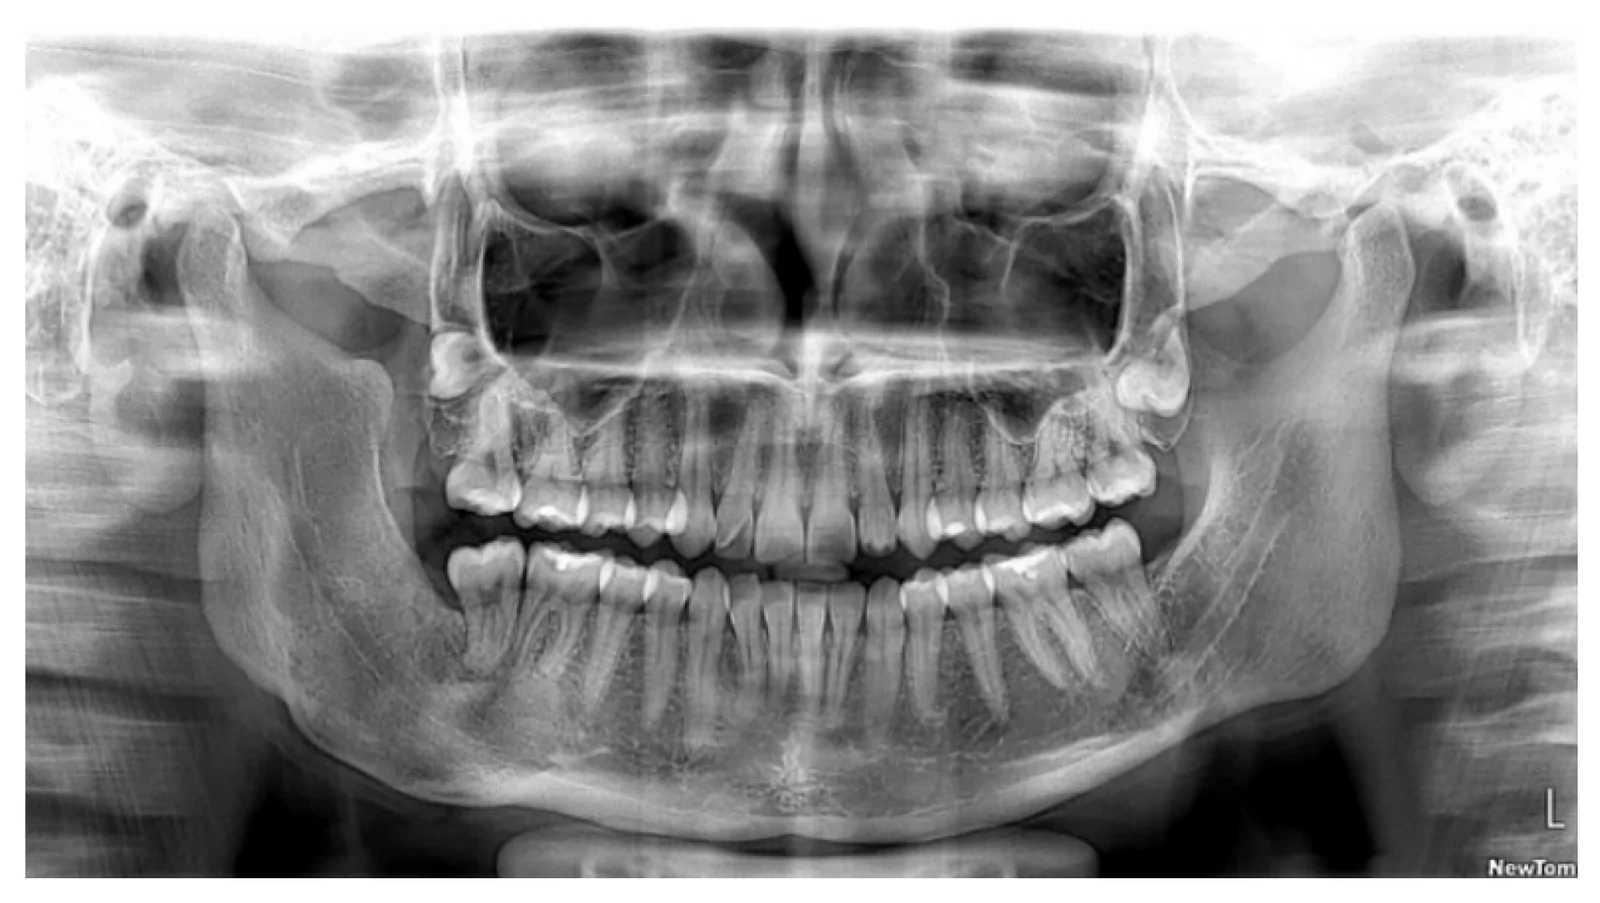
Panoramic radiograph at 2-year follow-up, showing maintained bone regeneration without evidence of recurrence or residual pathological tissue.
